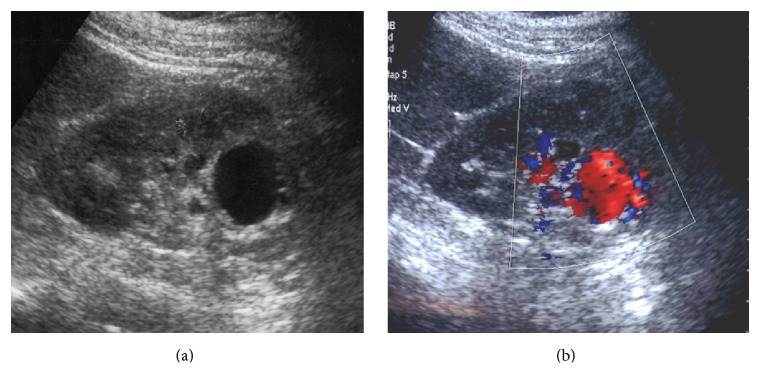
Vascular malformation. Longitudinal gray scale US (a); color Doppler US (b). Gray scale US of the right kidney shows an anechoic renal lesion. At color Doppler vascular flow is depicted within the lesion allowing the diagnosis of a vascular malformation.
